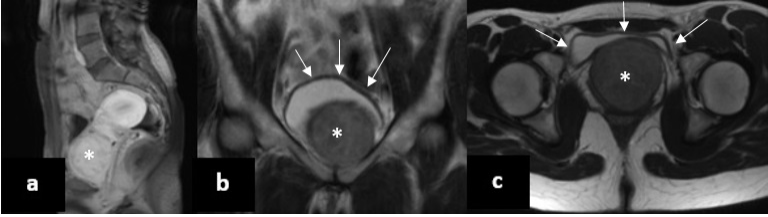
(# Case 1) MRI scan showing the exact location of the leiomyoma at the anterior vaginal wall at (a) sagittal (T1- weighted), (b) frontal and (c) transverse planes (T2-weighted). The leiomyoma is indicated with an asterisk (*), while the circumference of the urinary bladder with multiple arrows.
